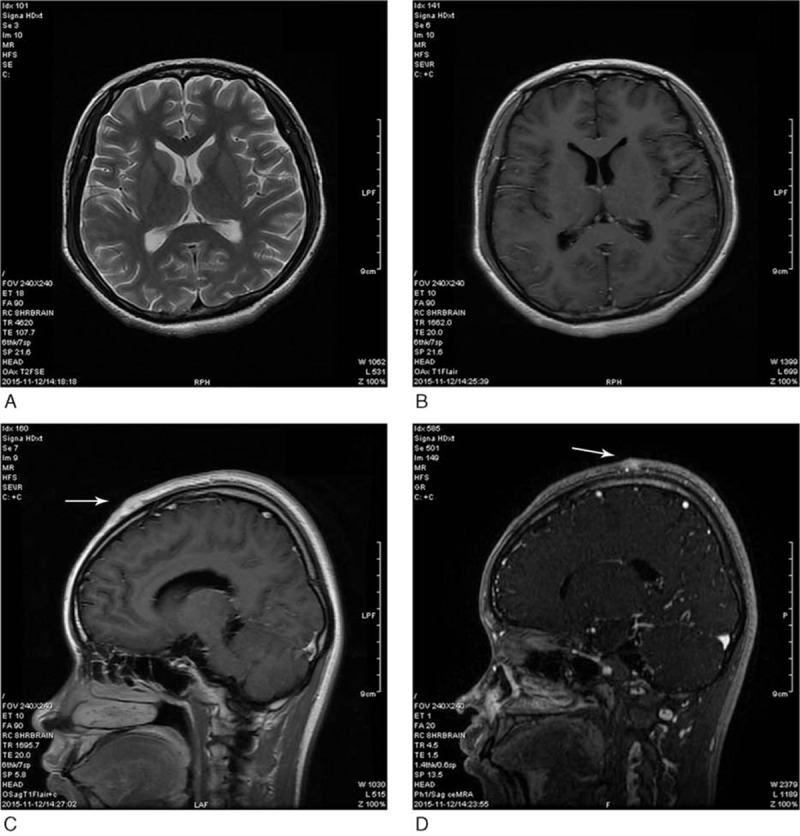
Cranial magnetic resonance imaging scans (A, B, C, D) showing no abnormalities. The arrows show a suspicious enhanced signal in the frontal and parieto-occipital regions.
